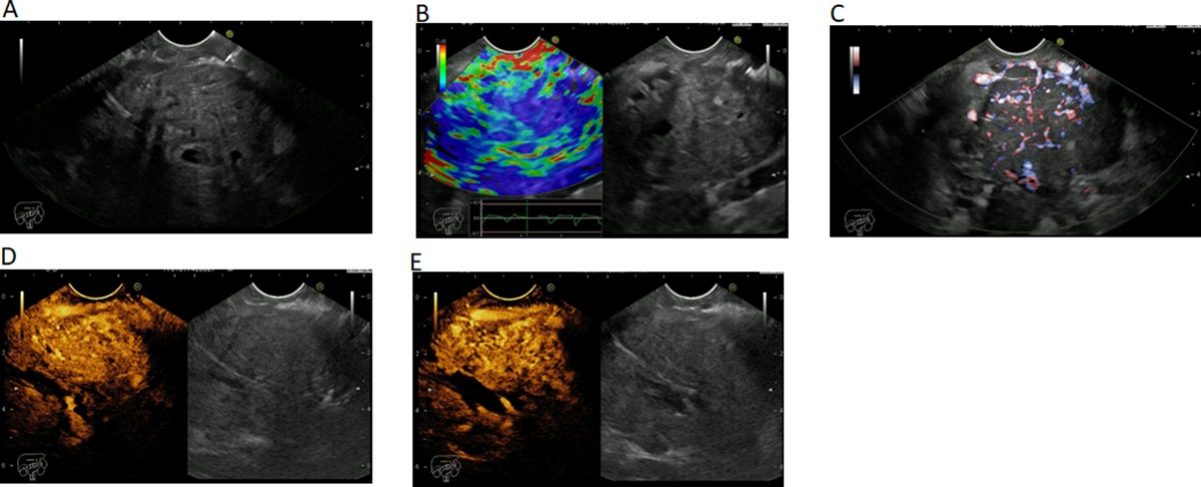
EUS imaging of a neuroendocrine tumor in (A) gray scale. (B) elastography. (C) color Doppler. (D) contrast enhancement–arterial phase. (E) contrast enhancement–venous phase.
